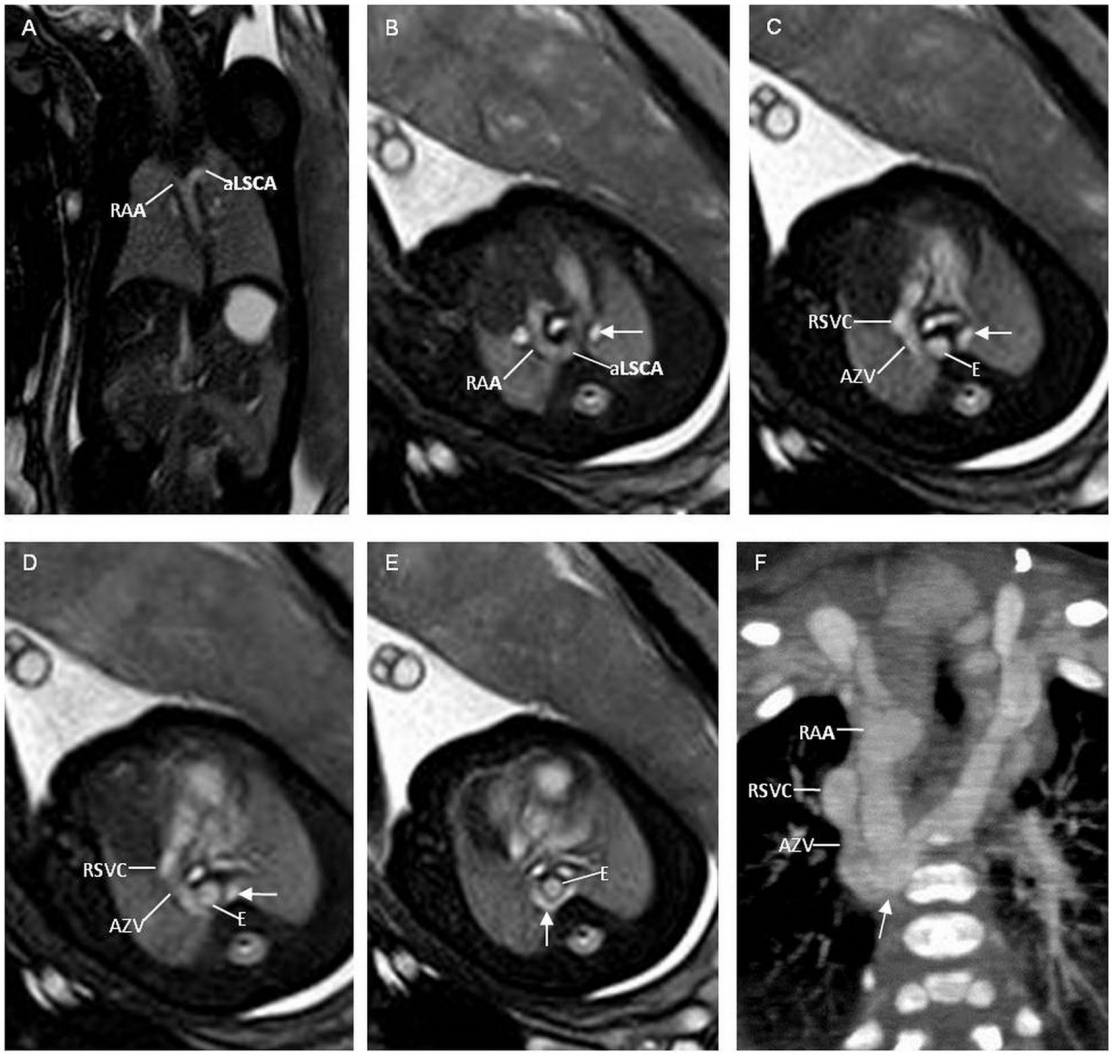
A 33-week fetus with a retroesophageal left brachiocephalic vein (LBCV) and a right aortic arch with aberrant left subclavian artery (RAA/aLSCA). Fetal CMR coronal view image of the aortic arch and sequential transverse view images show a right aortic arch (RAA in A and B) with an aberrant left subclavian artery (aLSCA in A and B), a LBCV (arrow in B), and a retroesophageal LBCV (arrows in C, D, and E) passing posterior to the esophagus (E in C, D, and E), joining the azygos vein (AZV in C and D), and finally draining into the superior vena cava (arrowheads in C and D); Postnatal CTA shows a right aortic arch (RAA in F) and a retroesophageal anomalous LBCV (arrow in F) joining the azygos vein (AZV in F) and finally draining into right superior vena cava (RSVC).
